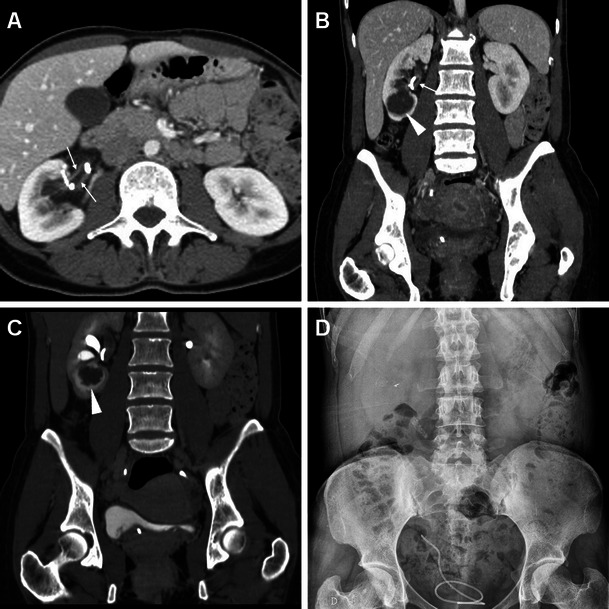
A 38-year-old female with intermittent fever and elevated C-reactive protein 2 weeks after PCNL treatment for right-sided nephrolithiasis. Contrast-enhanced MDCT with the ureteral stent in place showed absent hydronephrosis, mild enhancing ureteral thickening (*thin arrows in A, B*) indicating infectious pyeloureteritis and a 3-cm hypoattenuating lesion with peripheral delayed enhancement (*arrowheads in B and C*) at the lower renal pole consistent with an abscess. The patient recovered after intensive antibiotic treatment. During follow-up, distal stent migration was detected radiographically (D)
